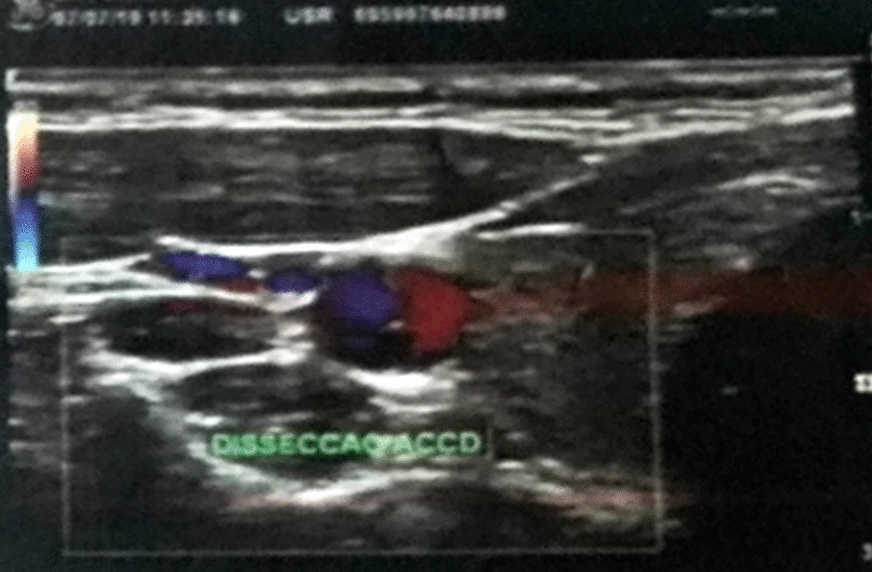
Ecocollordoppler image of the right common carotid artery. The exam demonstrated dissection of the vessel’s intimal layer, creating a false lumen
